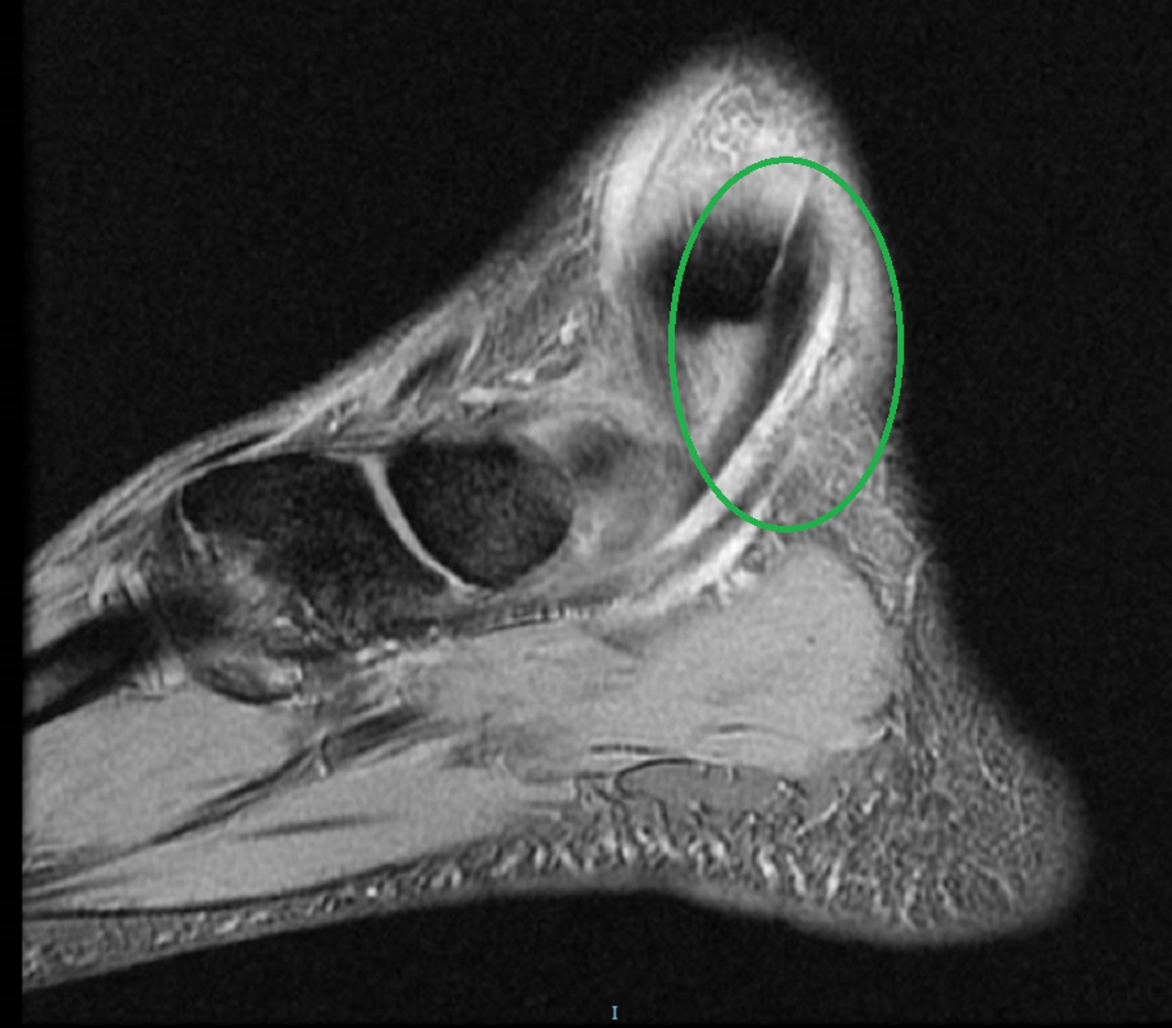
T2-weighted MRI (sagittal plane) demonstrating an anterior subluxation of the posterior tibialis tendon (green circle) over the medial malleolus with mild hypertrophy of the tibialis posterior tendon.
